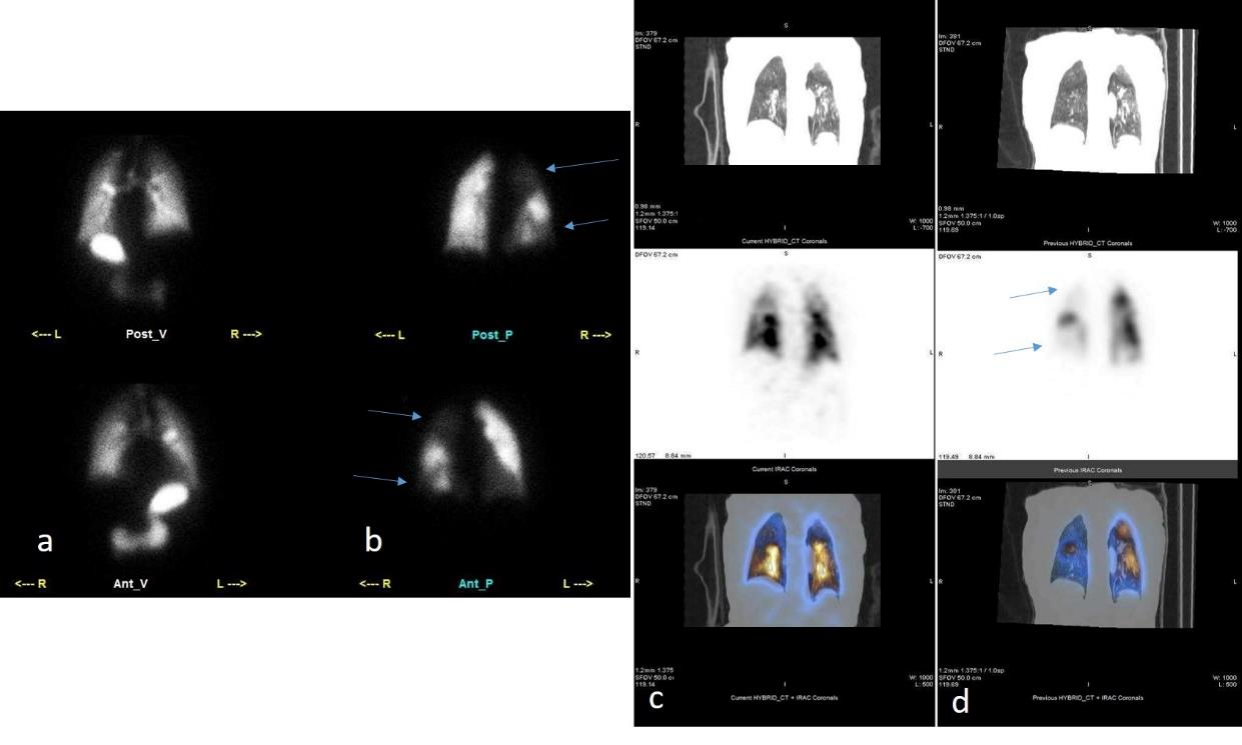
Lung planar V/Q images (a and b) and SPECT/CT V/Q images (c and d) of a patient. Multiple mismatched perfusion/ventilation defects are visible in the right lung, which are highly indicative of PE. The blue arrows point to the specific areas where these defects are observed, highlighting the regions of abnormal perfusion that do not correspond with ventilation, characteristic of PE
